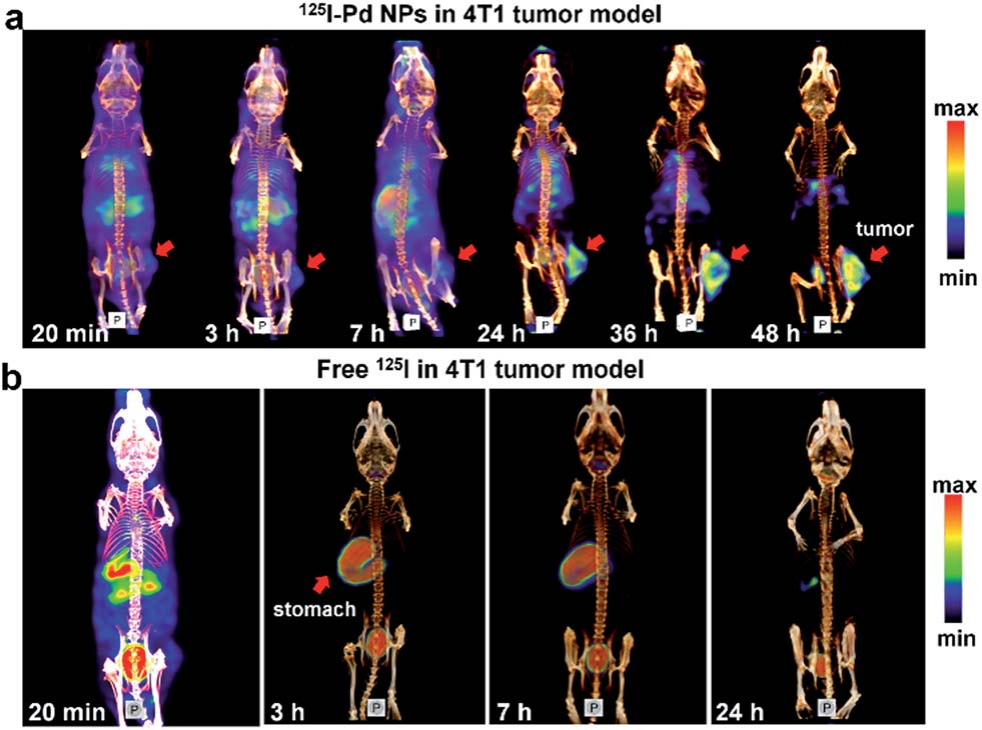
(a) SPECT/CT images of ^125^I–Pd–PEG in a subcutaneous 4T1 tumor model. (b) SPECT/CT images of Na^125^I in 4T1 tumor models at different times.
